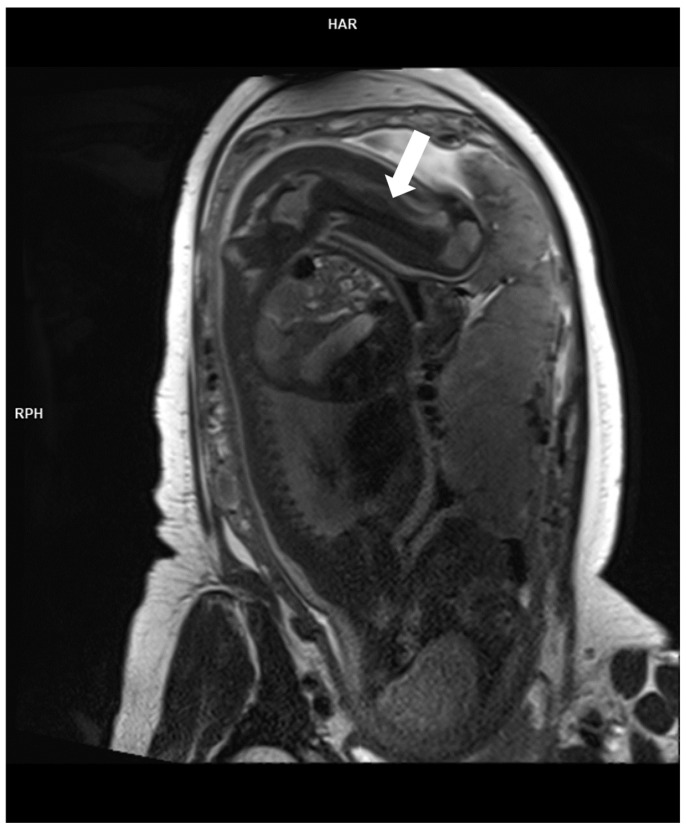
T2W HASTE image of fetal MRI at 34th week of gestation with femur (white arrow) imaged in profile. This image demonstrates the excellent contrast resolution of fetal MRI, enabling evaluation of morphology, mineralization, and cartilaginous components of the skeleton.
